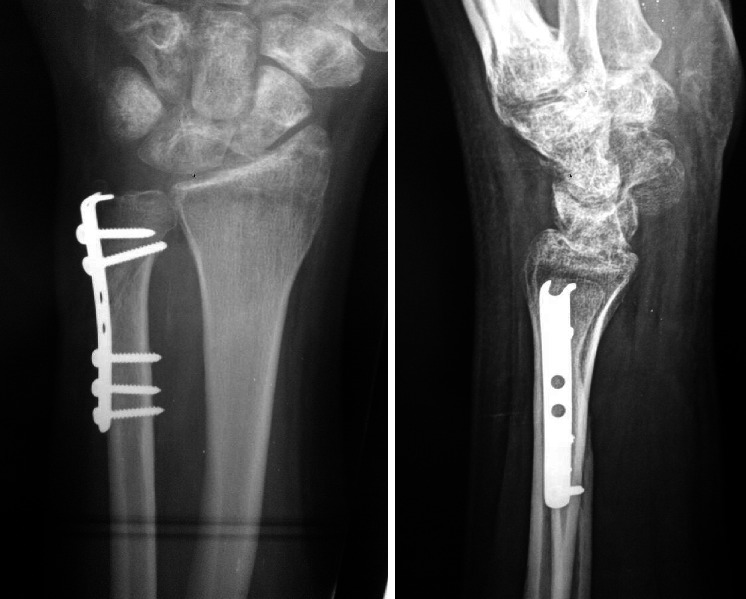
X-ray wrist anteroposterior and lateral views show follow-up of the patient after union.
